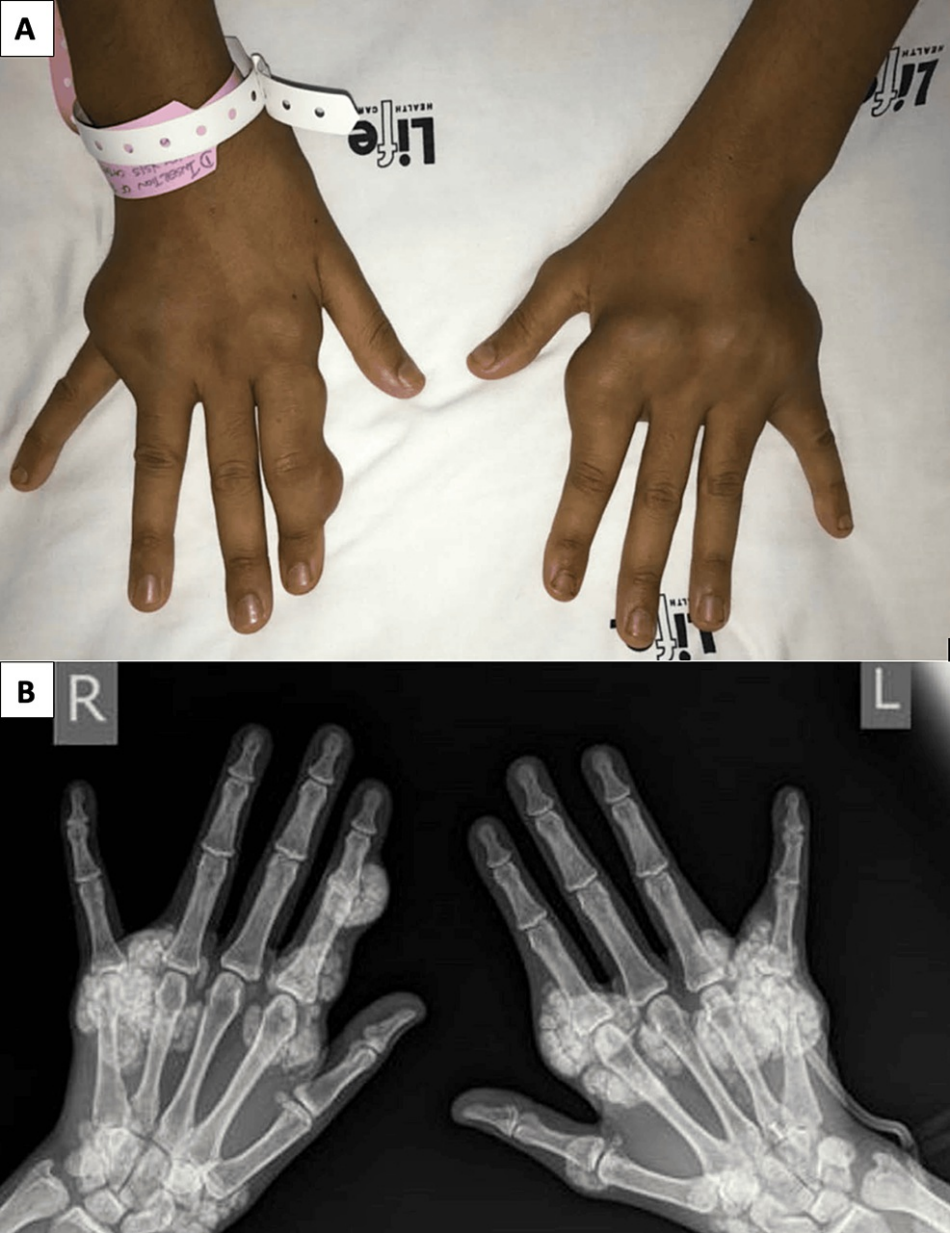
Metastatic calcinocis cutis (A) Nodules predominantly affecting the metacarpophalangeal joints of both hands. (B) Plain radiograph showing periarticular carpal, metacarpophalangeal joints, and subcutaneous calcified nodules without bony erosions.
